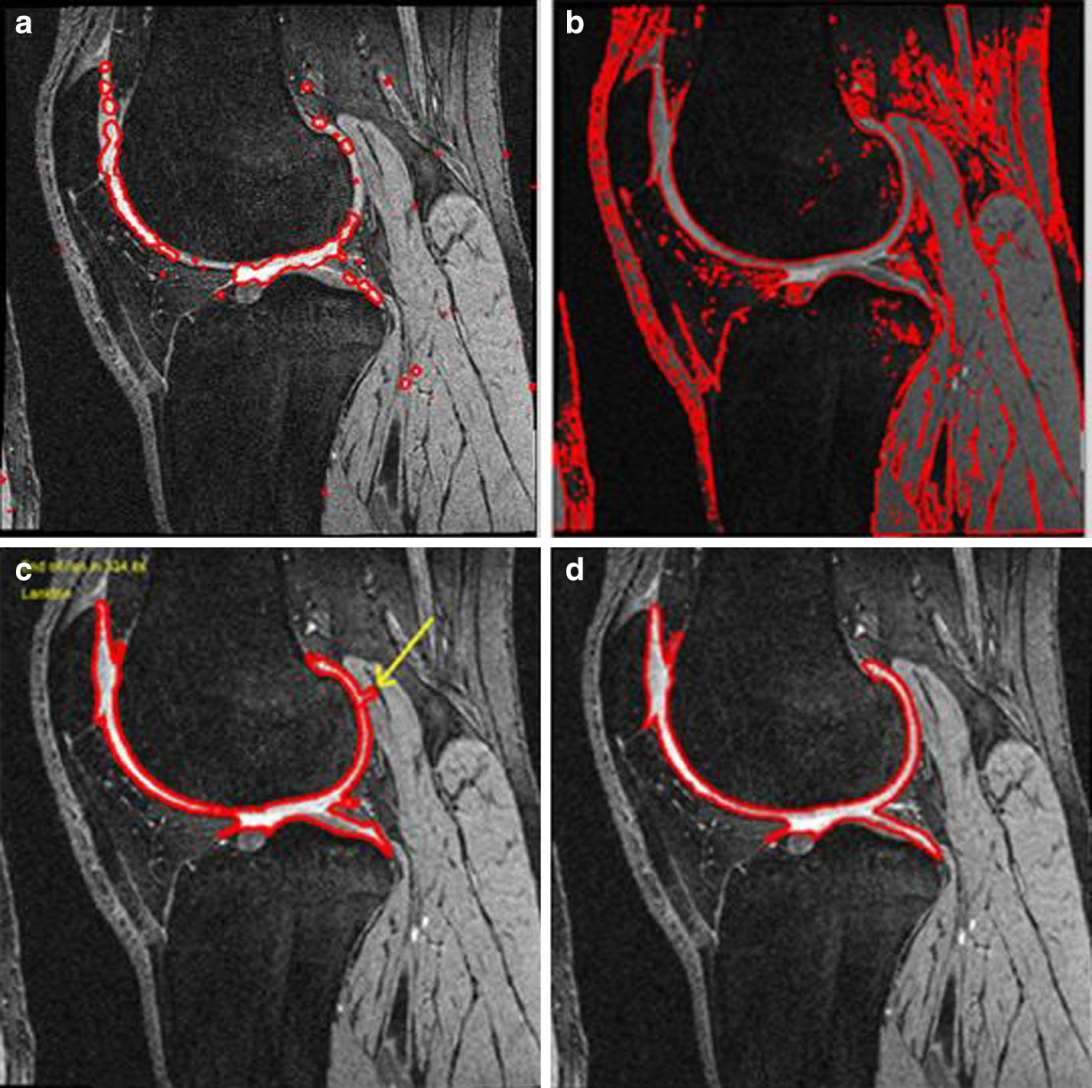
Cartilage segmentation in knee MRI. a Edge-based level set based cartilage segmentation in knee. b Global region region-based level set. c Localizing region-based level set. d Referenced manual segmentation
